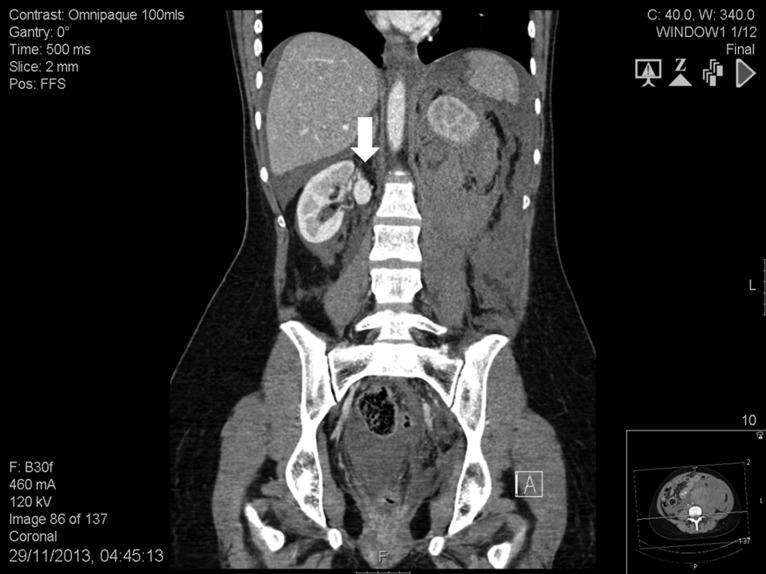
Abdomen contrast-enhanced computed tomography scan showing an intact 2.2-cm right renal artery aneurysm (white arrow).
